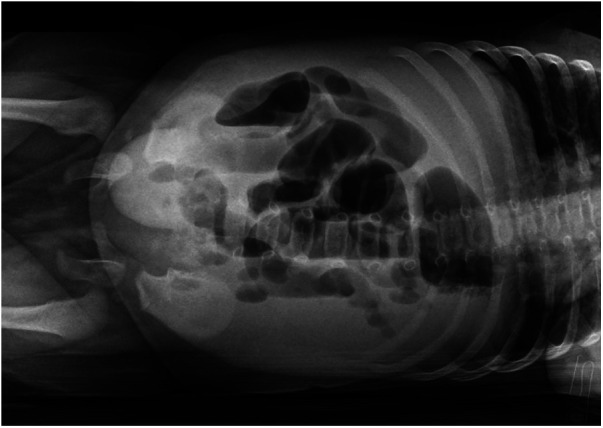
Direct abdominal x-ray performed after clinical worsening suggesting intestinal occlusion with multiple aereal levels and hygro-gas stasis.
